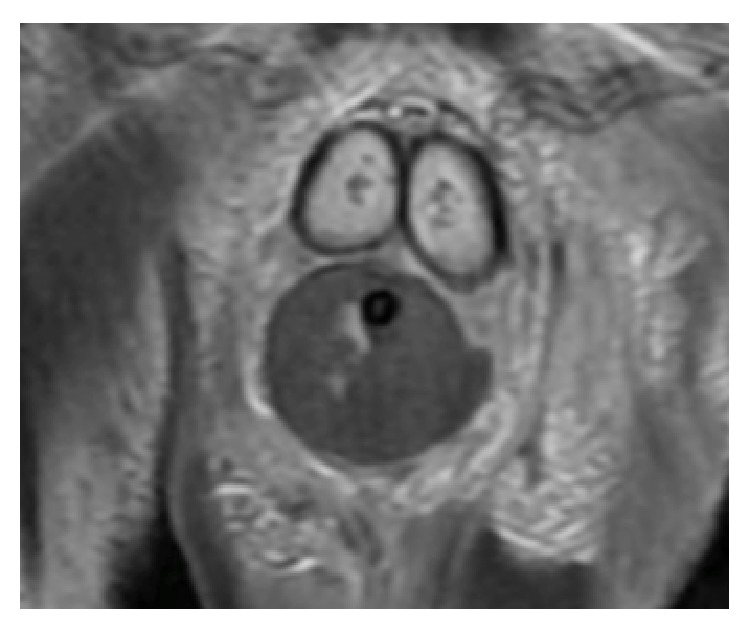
A representative MRI T1 image after gadolinium showing a coronal cut of the base of the penis with both corpora cavernosa having high signal intensity due to gadolinium uptake whereas the corpus spongiosum does not uptake gadolinium.
